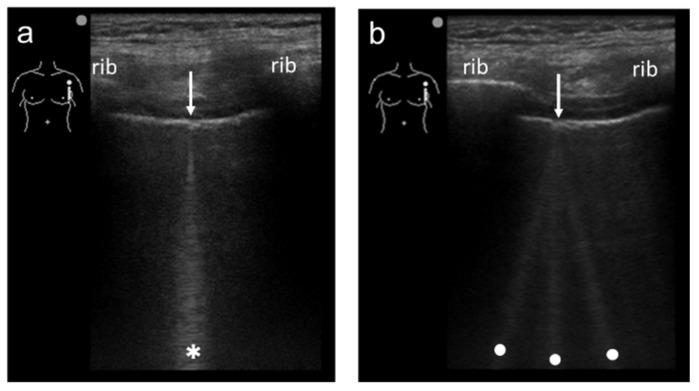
Ultrasound images obtained by MicroMaxx scanner (SonoSite) with a linear transducer without (a) and with spatial compound imaging (b). A focal point was set as default by the manufacturer, and it is not shown on the screen. With spatial compound imaging enabled, single B-line (asterisk) changes to multiple lines (dots) radiating from the same point (arrows) on the pleural line.
